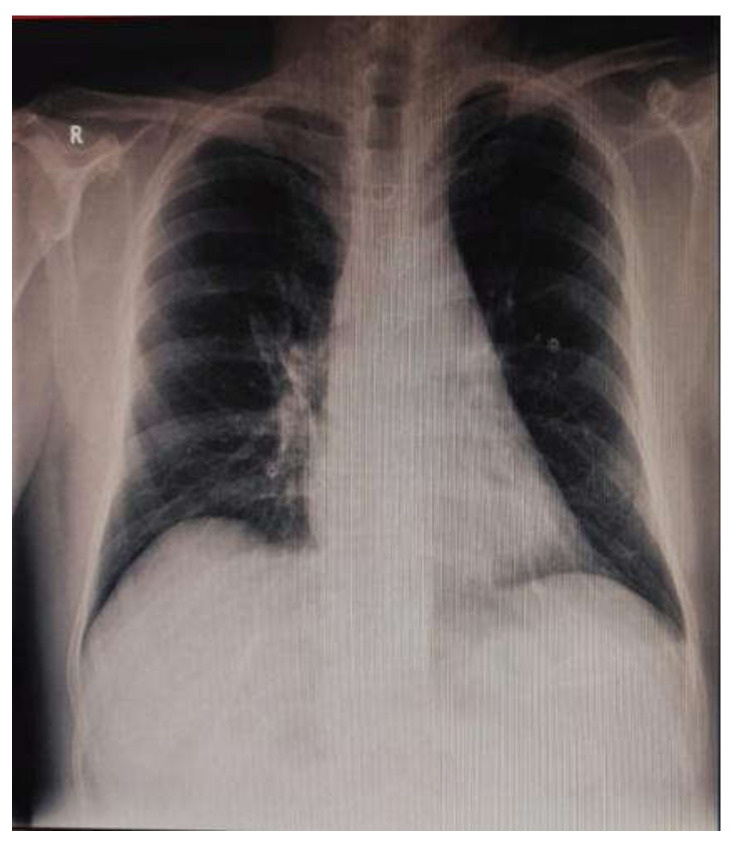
Postoperative chest X-ray.
